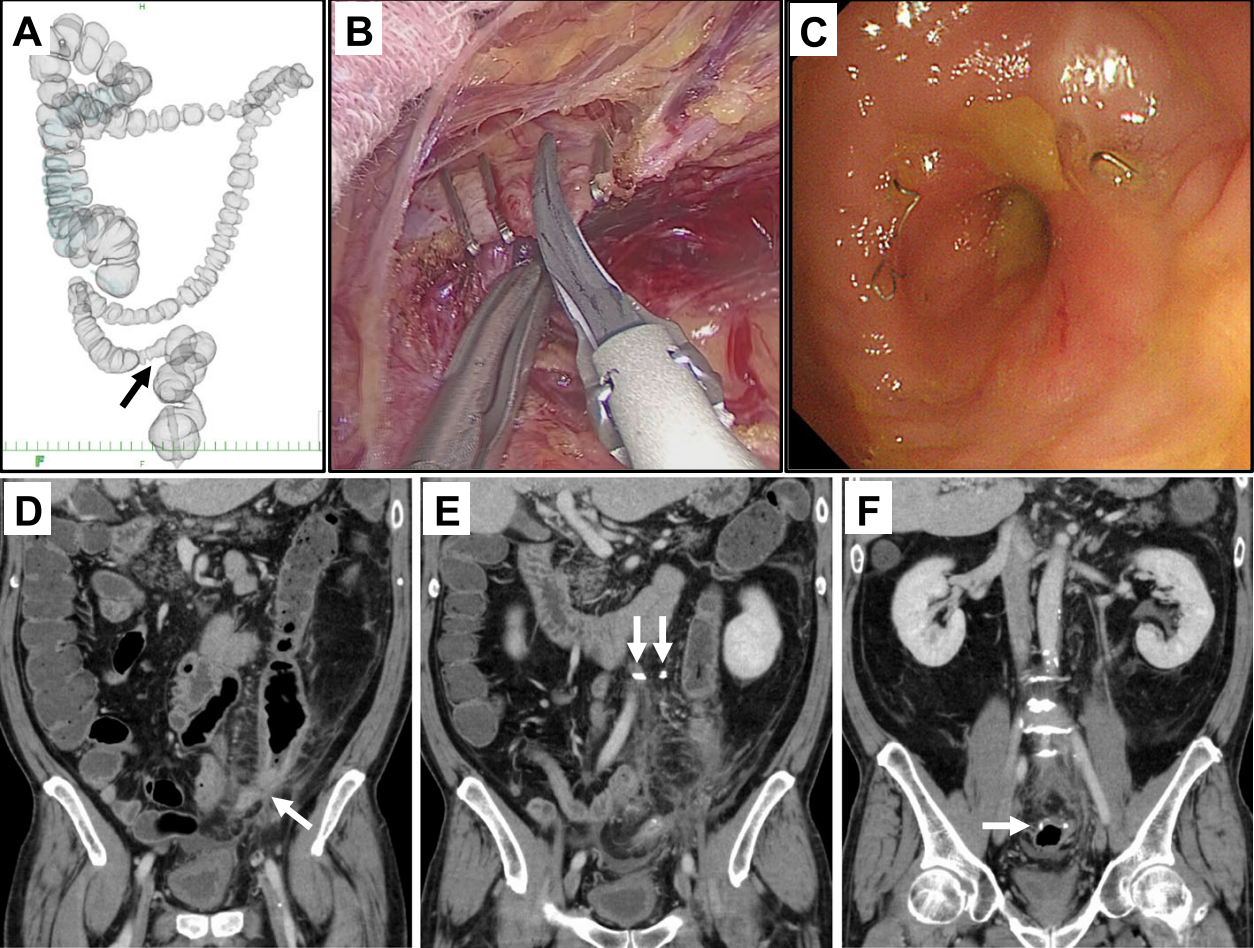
Case 1: a case of upper rectal cancer with postoperative stenosis of the reconstructed colon. a Preoperative computed tomography (CT)-colonography shows the PDM. The black arrow shows sigmoid colon cancer. b During laparoscopic surgery, the root of the inferior mesenteric artery (IMA) was cut. c Colonoscopy shows postoperative stenosis of the reconstructed. d–f CT shows colonic dilatation caused by stenosis of the reconstructed colon. d The white arrow shows colonic stenosis and dilatation of the oral side. e The white arrows show the clips on the IMA (left) and left colic artery (LCA) (right). The contrast enhancement at the distal side of the reconstructed colon was weak. f The white arrow shows the staple line at the anastomosis
